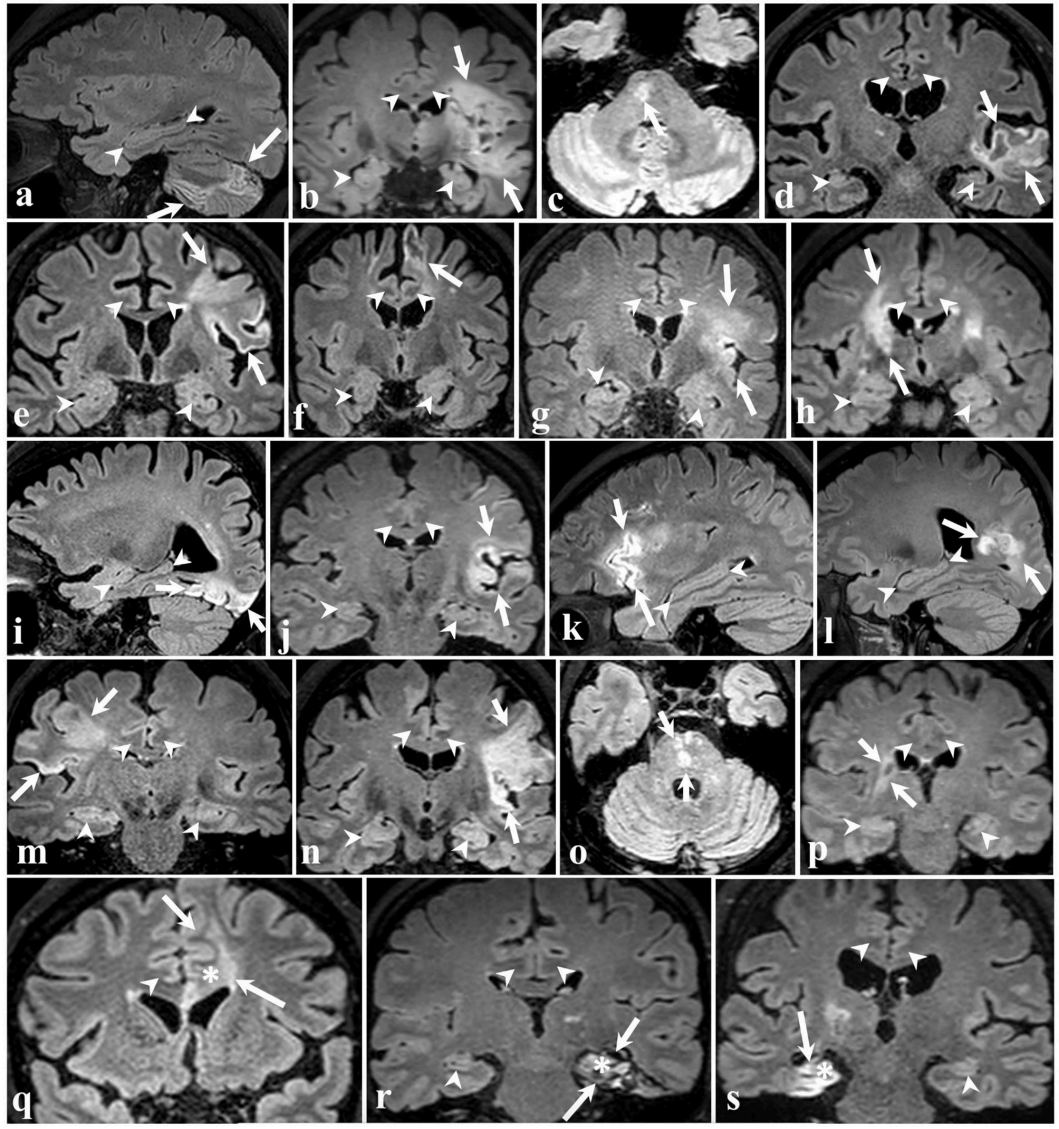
3D FLAIR brain images of all 19 participants in different planes. The arrows point to ischemic lesions and the arrowheads demarcate some of the limbic structures. In 16 patients (a–p) shown in the four top rows, the stroke did not affect limbic structures. The bottom row shows three cases wherein ischemia injury involves the left cingulate gyrus (q), left hippocampus (r), and right hippocampus (s), marked by an asterisk.
